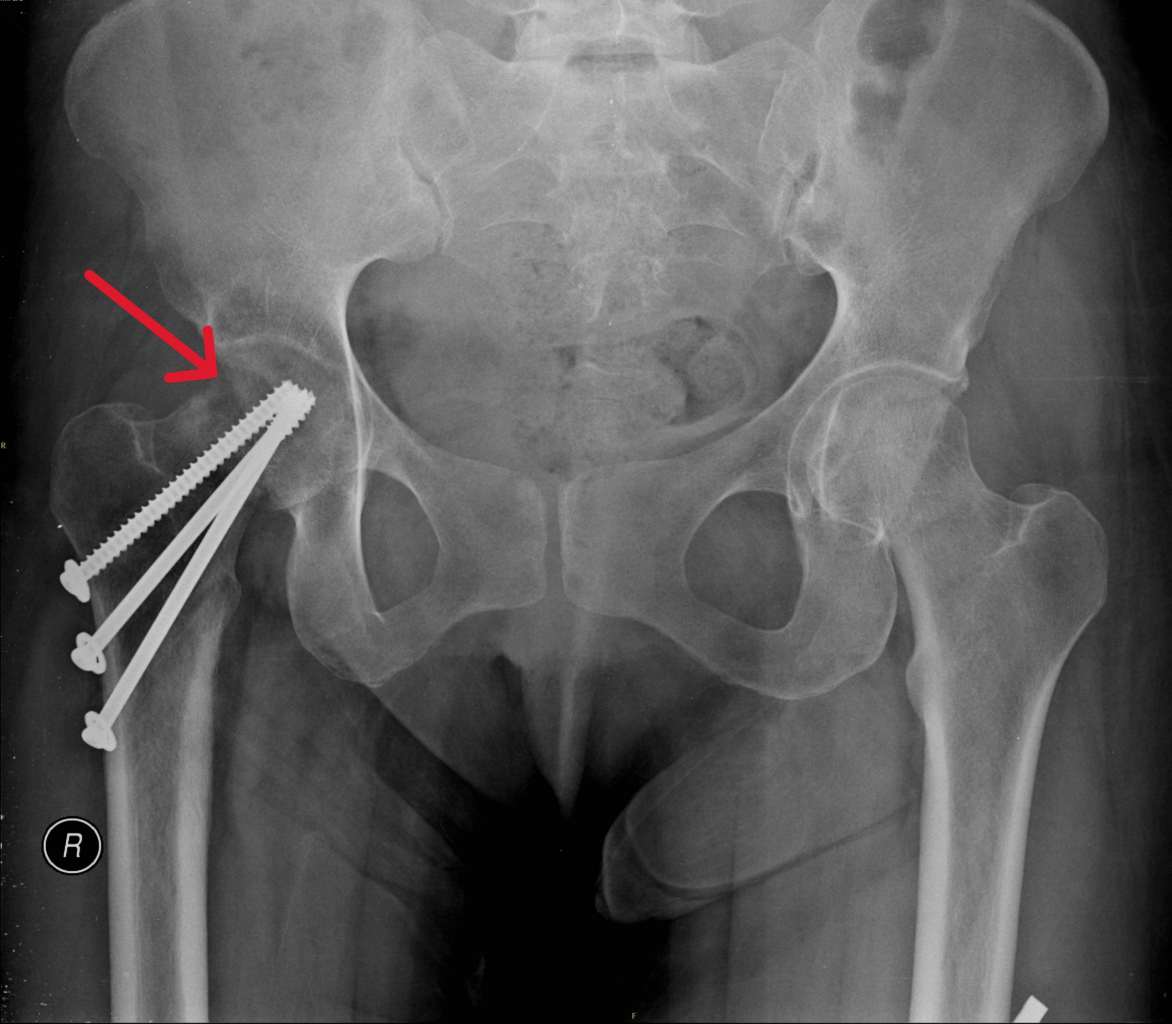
Pelvic X-ray at one-year post-op The red arrow shows the area of radiolucency at the previous fracture site.
